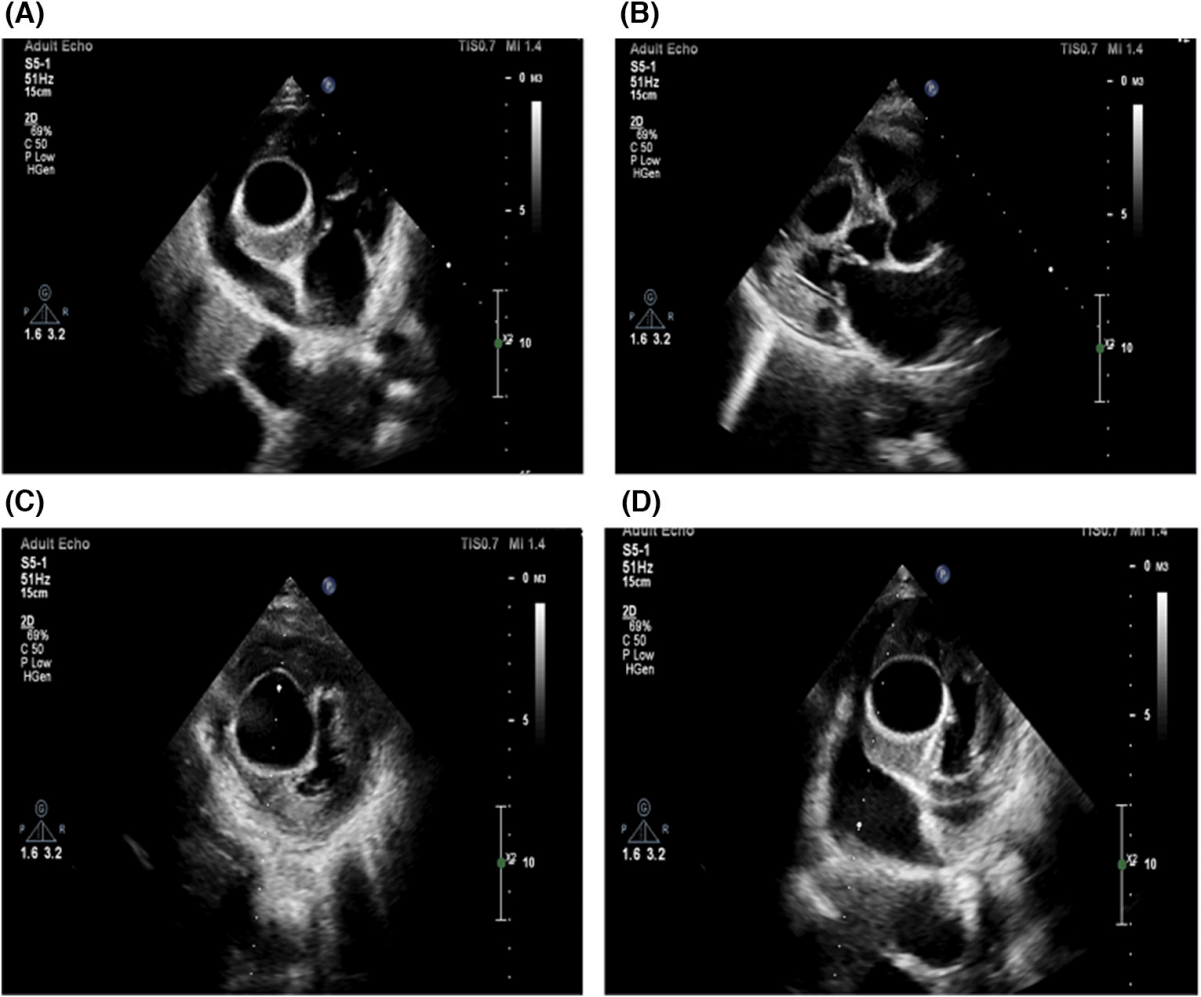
Transthoracic echocardiography shows (A, D) in a four‐chamber view a large, well‐defined intramyocardial cystic lesion (with no obvious septation) in the mid part of the septum with mild compression effect on RVOT without gradient. (B, C) The parasternal long axis and short axis view revealed a cystic lesion.
